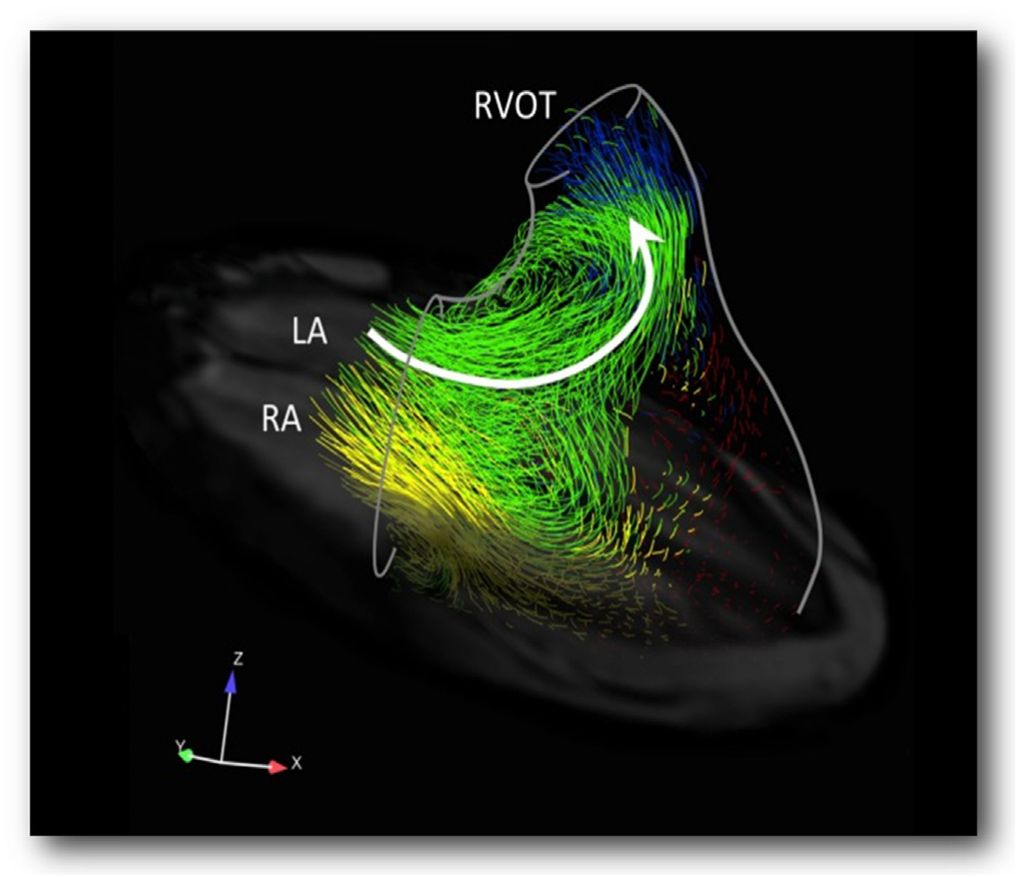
Normal RV flow patterns as visualized by 4D flow magnetic resonance imaging. Pathline visualization of RV blood flow during late diastolic filling in a 70-year-old healthy individual with a left ventricular end-diastolic volume index of 61 mL/m^2^. The inflowing blood is channeled toward the outflow tract through the ring vortex (indicated by the white arrow). Different flow components are represented as follows: direct flow (green), retained inflow (yellow), delayed ejection flow (blue), and residual volume (red). Some pathlines in the lateral region of the RV have been omitted to reveal those near the interventricular septum. A semitransparent 4-chamber image provides anatomical orientation. LA, left atrium; RA, right atrium; RV, right ventricle; RVOT, right ventricular outflow tract. Reproduced with permission from Fredriksson et al.^9^
